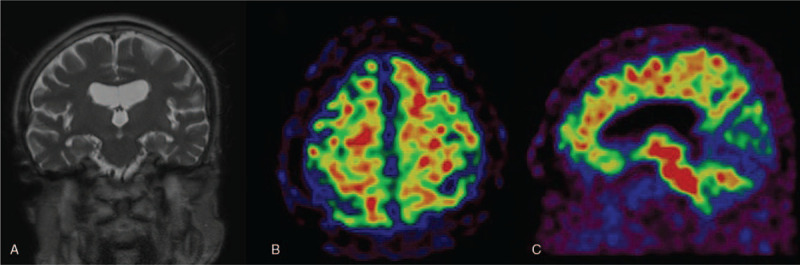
Brain MRI revealed bilateral medial temporal atrophy on coronal plane (A). ^18^F-flutemetamol PET scan demonstrated amyloid accumulation in bilateral frontal lobes (B) and precuneus (C). MRI = magnetic resonance imaging, PET = positron emission tomography.
